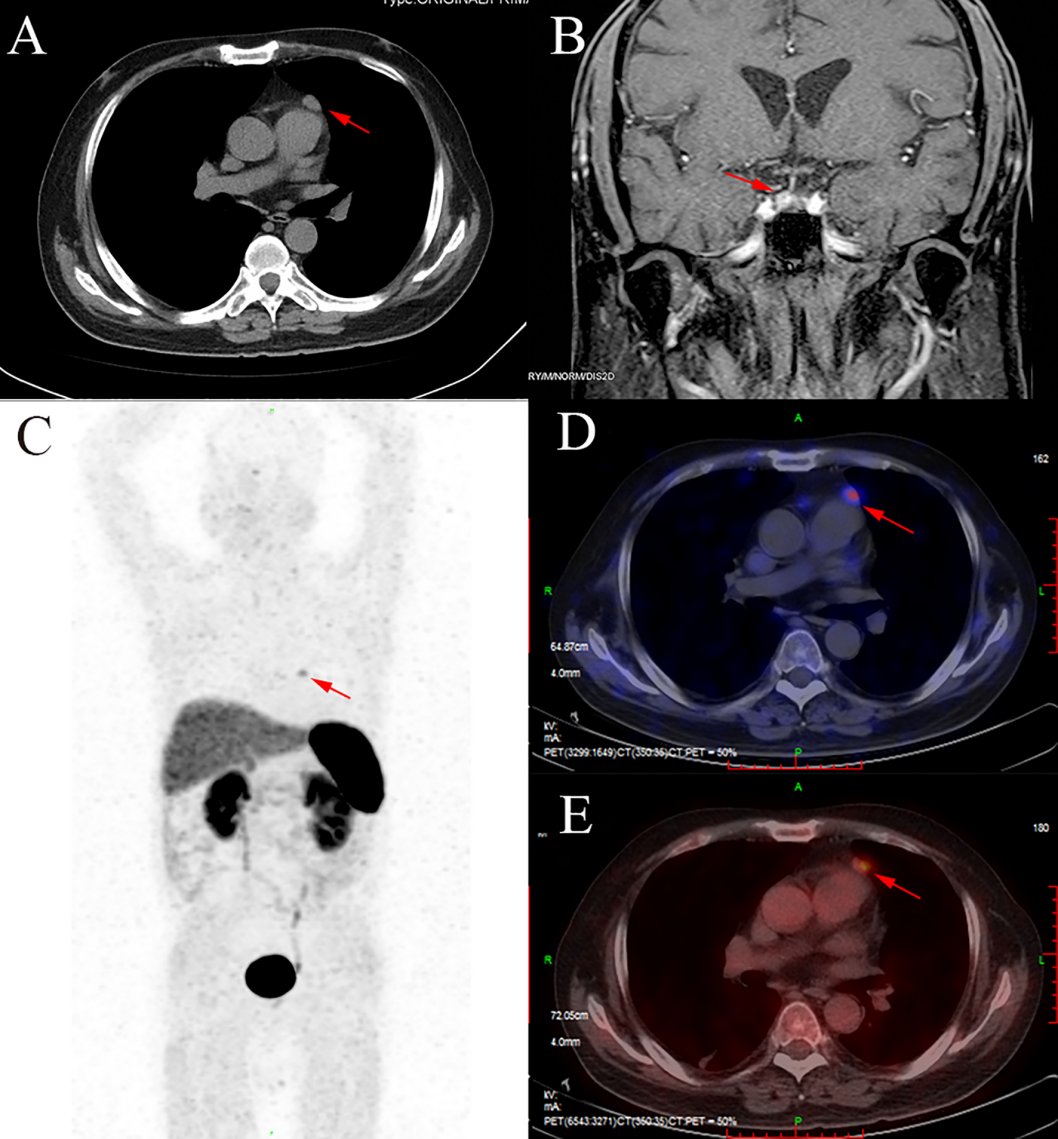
The CT, MRI, and PET/CT images of this patient. (A) A soft tissue density nodule in the mediastinum (red arrow). (B) A nodule in the right side of the pituitary (red arrow). (C, D) The anterior mediastinal nodule with increased ^68^Ga uptake (red arrow). (E) The anterior mediastinal nodule with increased fluorodeoxyglucose uptake (red arrow).
